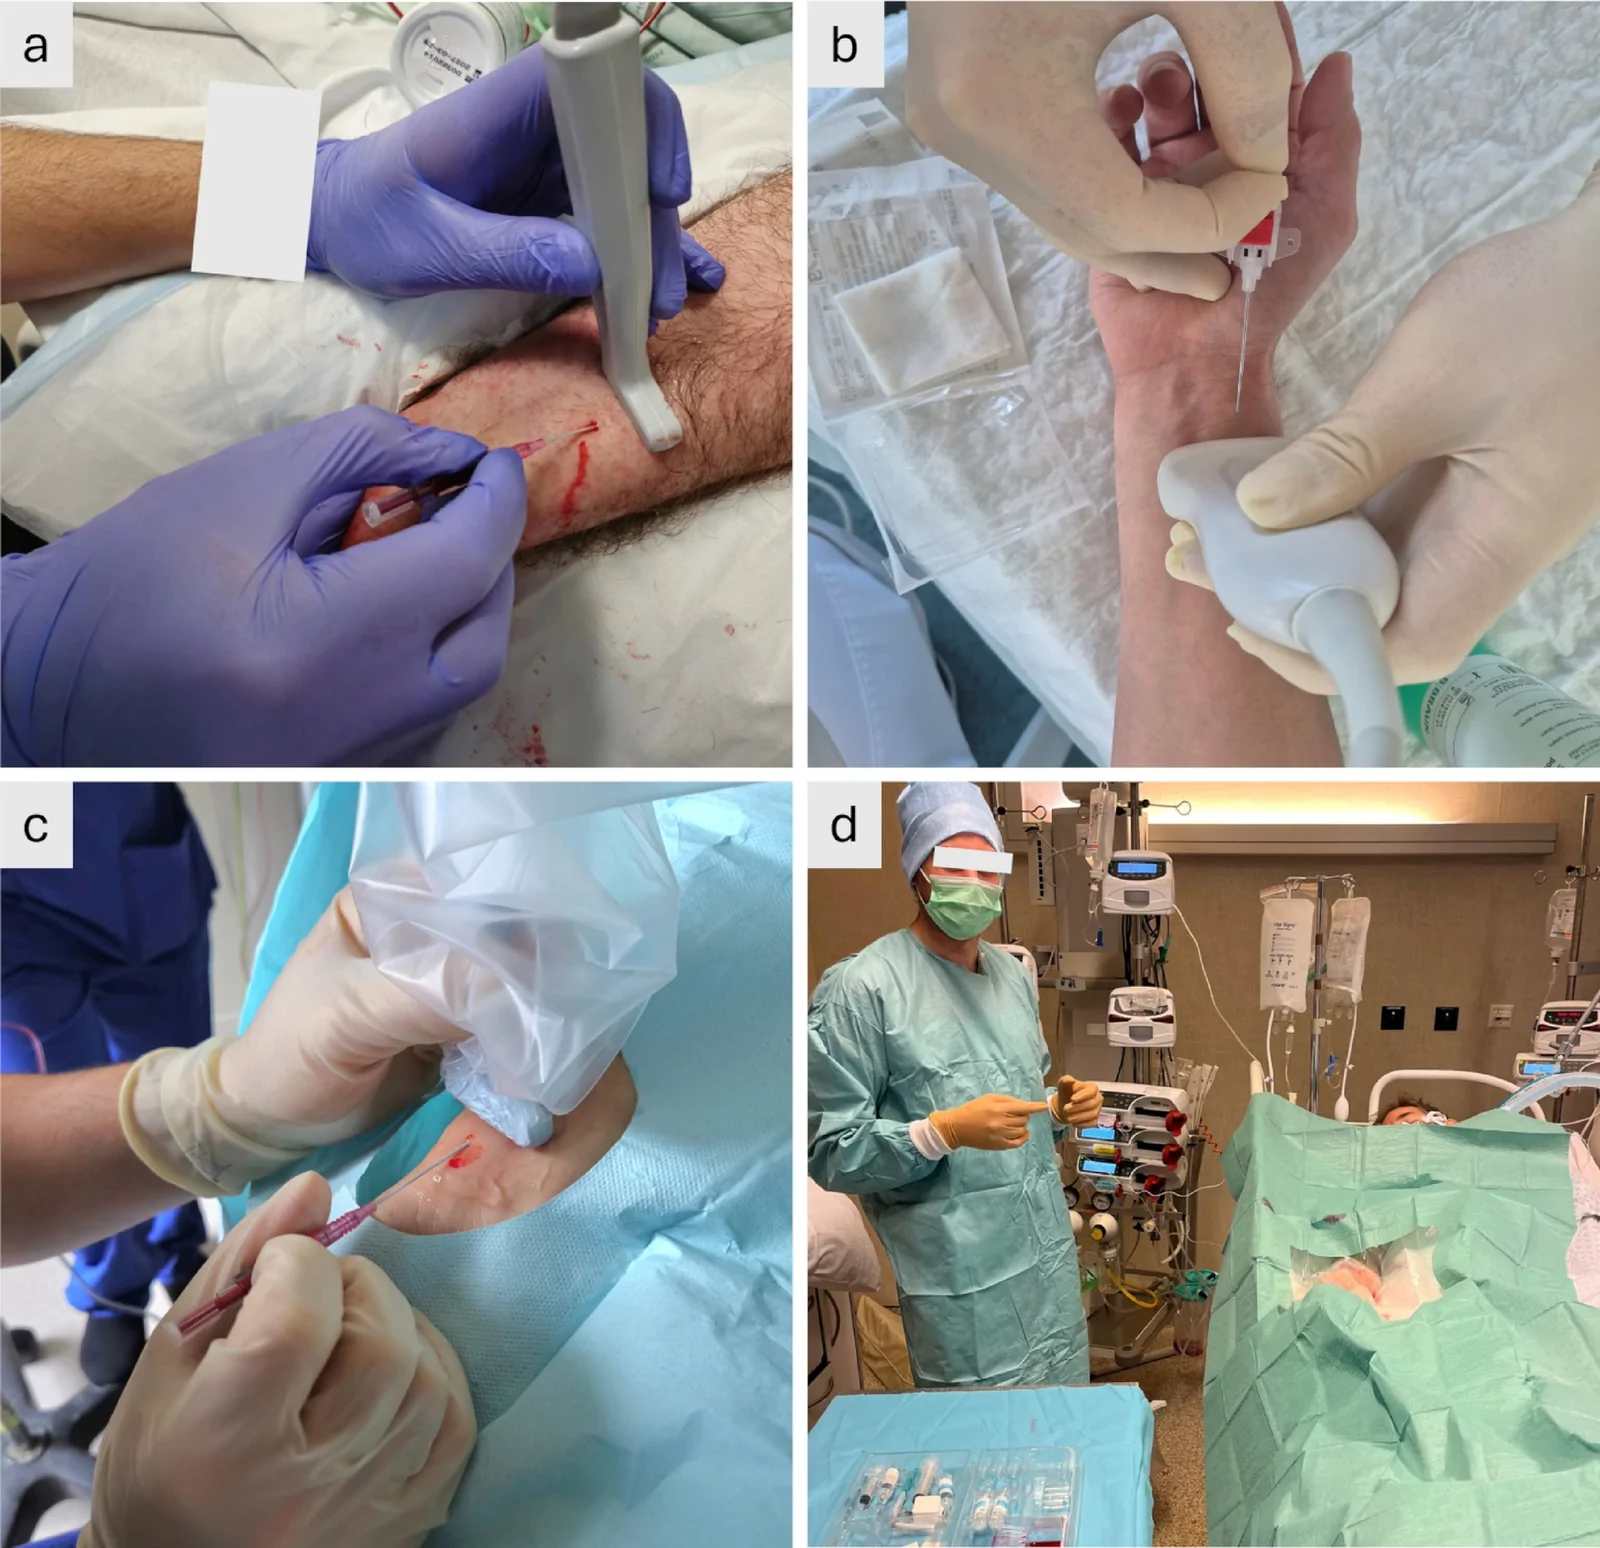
Spectrum of infection prevention practices in critical care units for insertion of radial catheters (a) catheterisation using an over-the-needle technique with ultrasound guidance using non sterile gloves (no insertion bundle) (b) catheterisation using an over-the-needle technique with ultrasound guidance using sterile gloves and an aseptic non-touch technique (ANTT) (c) catheterisation using an over-the-needle technique with ultrasound guidance using sterile barrier precautions (SBP) (d) catheterisation with the Seldinger technique without ultrasound guidance, using maximal sterile barrier precautions (MSBP)
